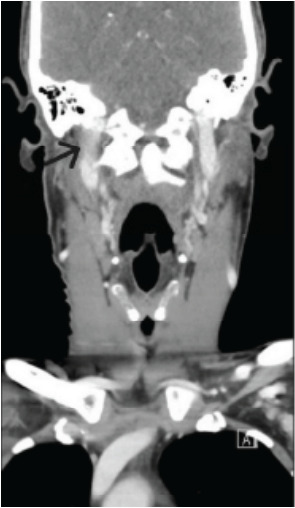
Coronal computed tomography with a black arrow demonstrating mild, asymmetric circumferential inflammation of the right carotid and extracranial internal carotid arteries.
